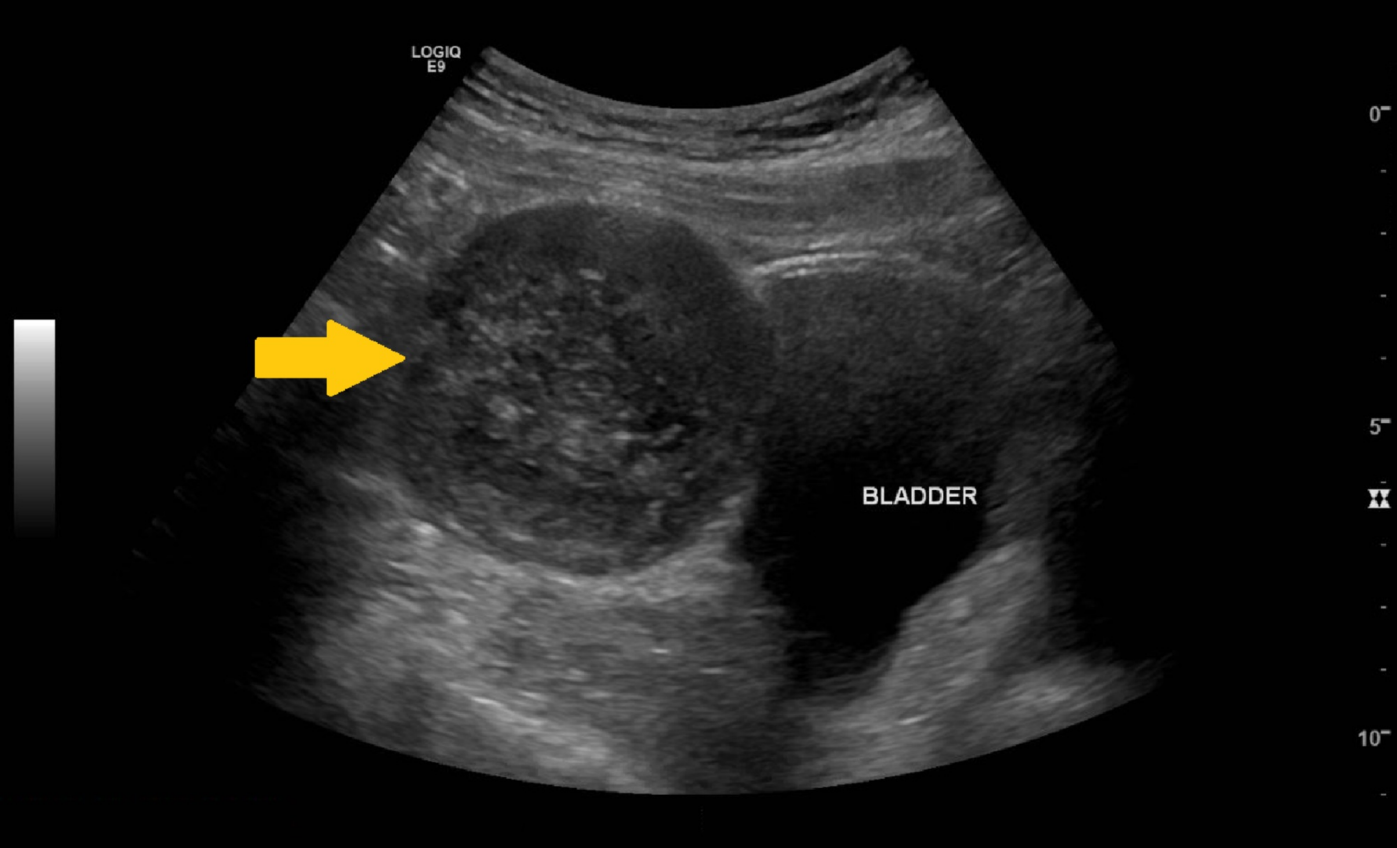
Retroperitoneal ultrasound demonstrating the pelvic mass located just superior to the urinary bladder (yellow arrow).
